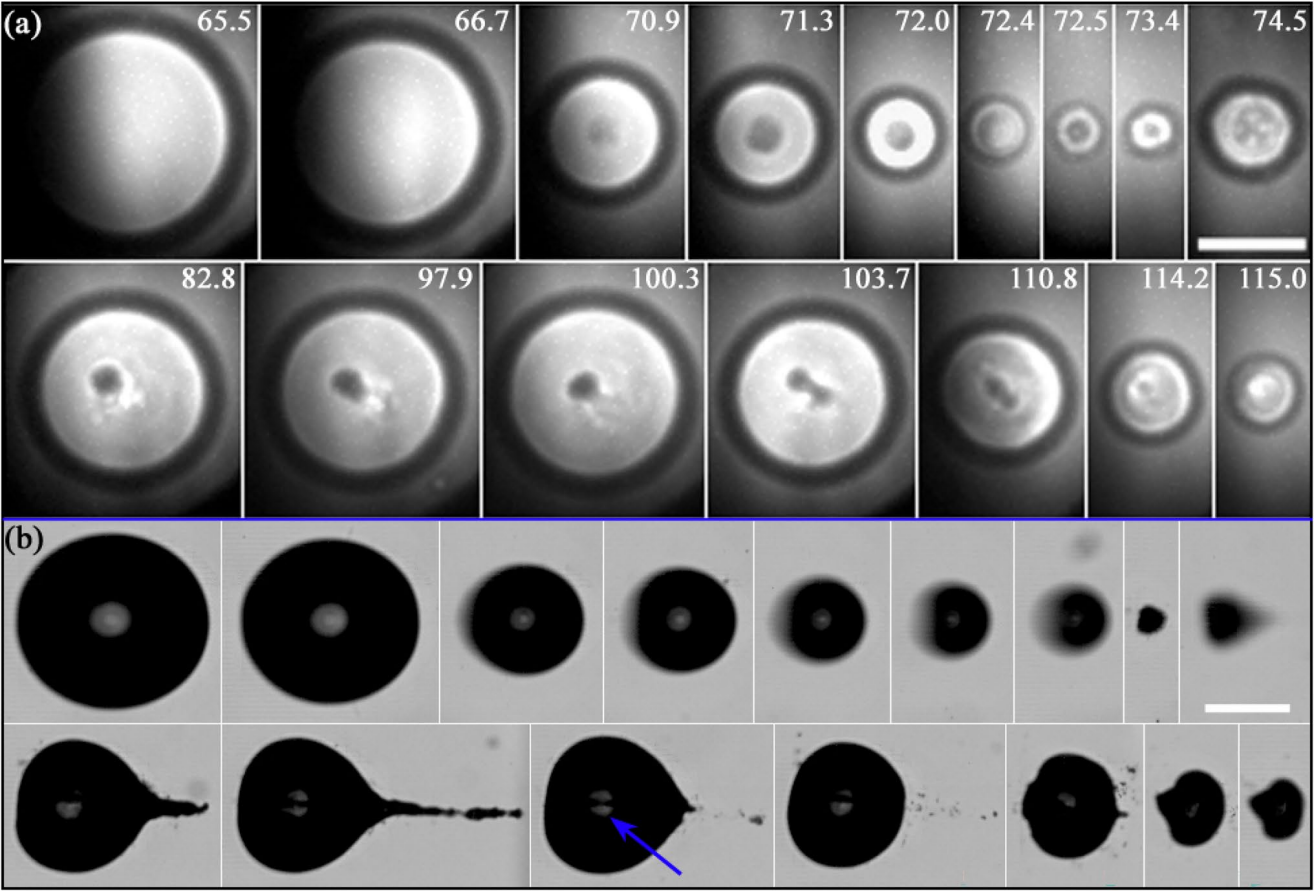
Bubble jetting at a stand-off distance of $$\gamma \simeq 2.5$$. a X-ray images acquired setting the glass disc normally to the beam direction as detailed in Fig. 1c, i.e. in the parallel view configuration. b Optical images taken from the side, perpendicular to the x-ray direction. The blue arrow highlights the partial visibility of the thin jet inside the gas cavity. The scale bars represent $$250\,{\upmu \text {m}}$$. The numbers indicate time in $$\upmu {s}$$
